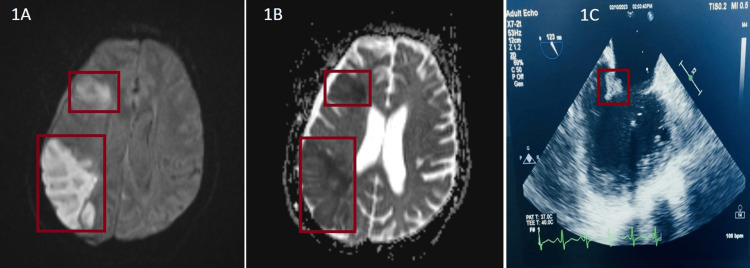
MRI brain and TOE findings 1A: MRI brain showing diffusion restriction in right frontal and parietal region suggestive of acute infarct. 1B: MRI brain showing low ADC suggestive of acute infarct in right frontal and parietal region. 1C: TOE showing verrucous mass under the surface of PML with absent independent motion ADC: apparent diffusion coefficient; MRI: magnetic resonance imaging; PML: posterior mitral leaflet; TOE: transoesophageal echocardiogram
